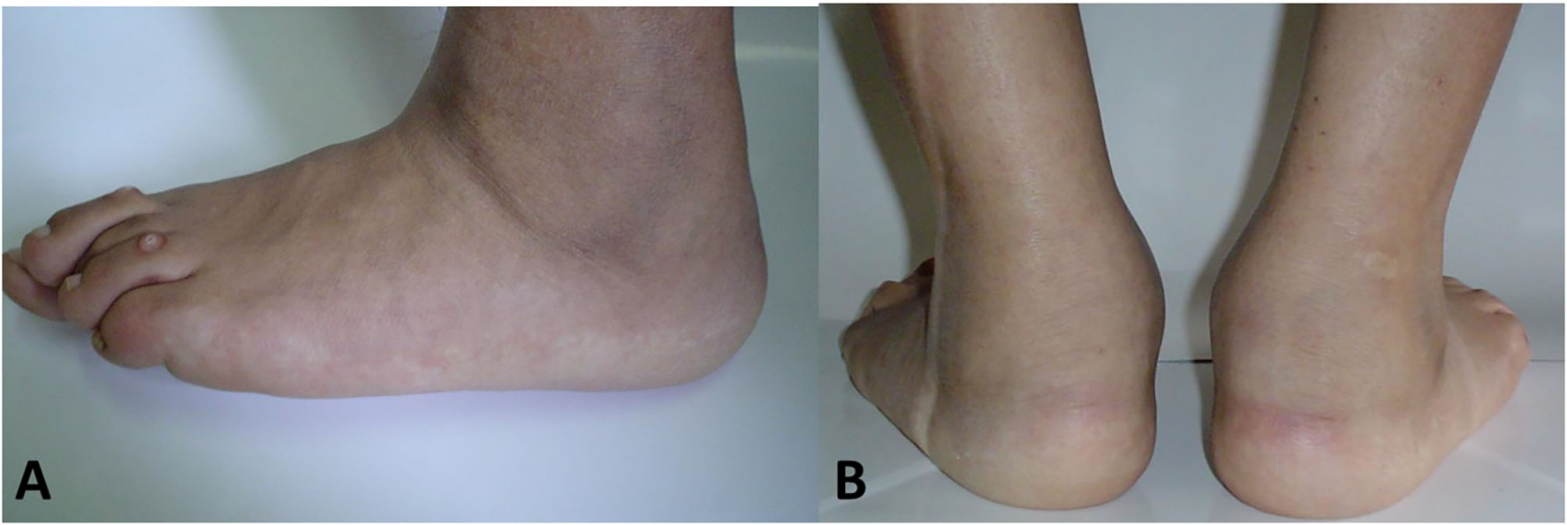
Lateral (A) and coronal (B) T-2 weighted-fat suppressed MR imaging showing edema in various tarsal bones, joint spaces and soft tissues in a 16 year old boy with chronic ankylosing tarsitis [Modified from Burgos-Vargas (46)].
